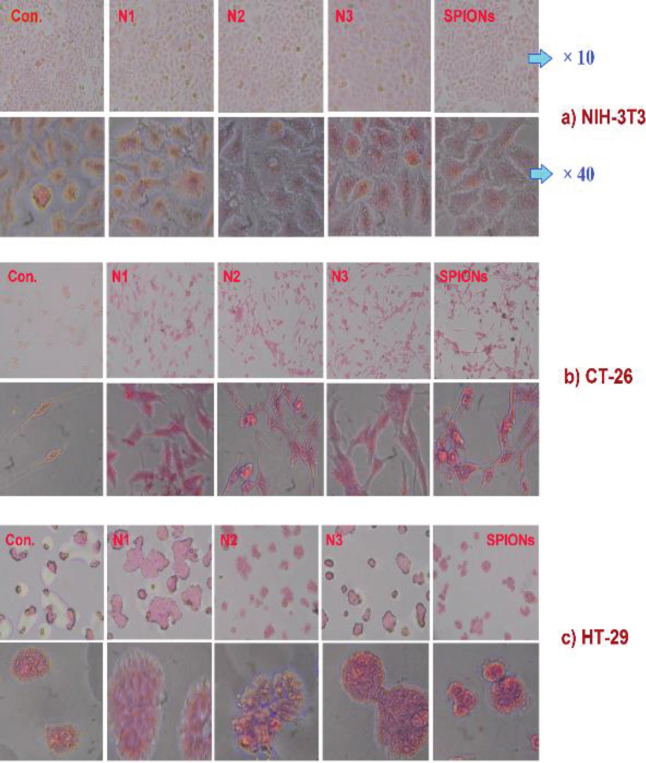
Confirmation of SPIONs and their derivatives around the cell membrane in CT-26 (b) and HT-29 (c) cancer cell lines relative to the normal NIH-3T3 (a) cell. (Control cells (Con.), Non-targeted nano-vector P@SPI (N1), targeted nano-vectors PS 5%-PNC@SPI (N2) and PS 10%-PNC@SPI (N3), and SPIONs)
